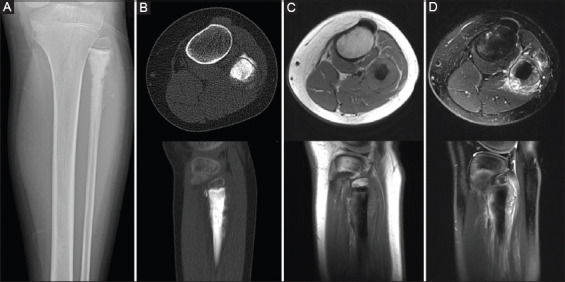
Exemplary radiographic imaging of an osteosarcoma in the left proximal fibula in a 10-y old boy (Table 2, case 9). (A) Plain radiograph shows “cloud-like” osteogenic destruction in medullary and cortical bone with Codman triangle and soft tissue mass in the proximal fibula. (B) Transverse (top) and sagittal (bottom) CT images add details of osteogenic destruction in the proximal fibula. Transverse CT image shows a lamellate reaction. (C) Transverse (top) and sagittal (bottom) T_1_-weighted MRI reveals a low signal intensity mineralized compartment in medullary and cortical bone and an intermediate signal intensity soft-tissue non-mineralized compartment around the proximal fibular tumor. (D) Transverse (top) and sagittal (bottom) T_2_-weighted MRI shows a low signal intensity mineralized compartment in medullary and cortical bone, a medium-high signal intensity soft-tissue non-mineralized compartment around the fibular tumor, and high signal intensity peritumoral edema.
